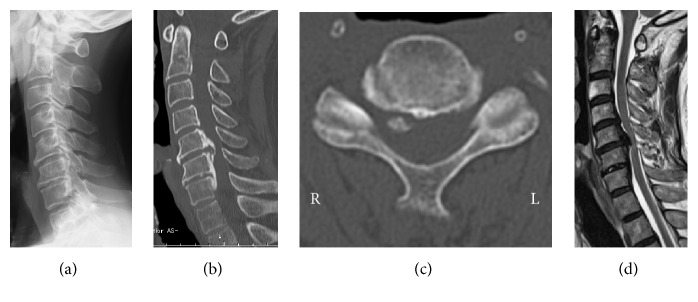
Preoperative radiological findings. (a) and (b): mixed type ossification of the posterior longitudinal ligament was observed on the lateral view of the cervical spine radiograph and sagittal view on computed tomography (CT). Alignment was lordotic and ossification did not exceed the “K-line.” (c): Foraminal stenosis was detected at bilateral C4/5 (1.5 mm on the left side and 2.5 mm on the right side) on axial view CT. (d): high-intensity areas in the spinal cord were not evident on magnetic resonance imaging.
